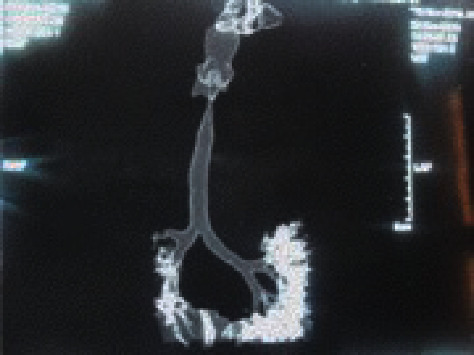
Subglottic stenosis with 3D reconstruction on a multidetector CT in an 18-month-old child with aquired subglottic stenosis.
